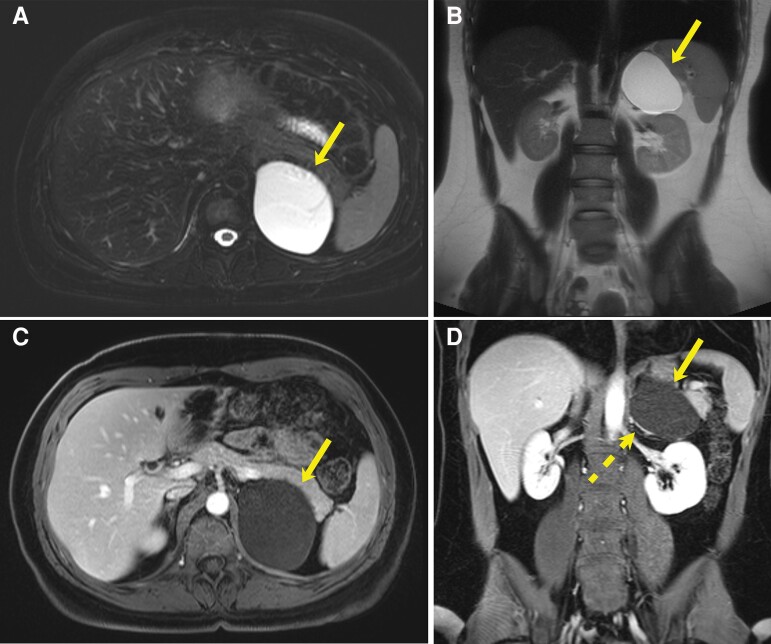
Diagnostic MRI showing a 7.4-cm simple-appearing left adrenal cyst with benign imaging features. (A) Axial T2-weighted fat-saturated, (B) coronal T2-weighted nonfat-saturated, and (C) axial and (D) coronal T1-weighted postgadolinium-enhanced MRI demonstrates a T1-hypointense, T2-hyperintense left suprarenal cyst (solid arrow) without any internal nodularity, septations, or enhancement. (D) The enhancing left adrenal gland along the medial aspect of the cyst demonstrating the “claw sign” with the cyst margin (dashed arrow), thereby confirming the cyst is adrenal in origin.
